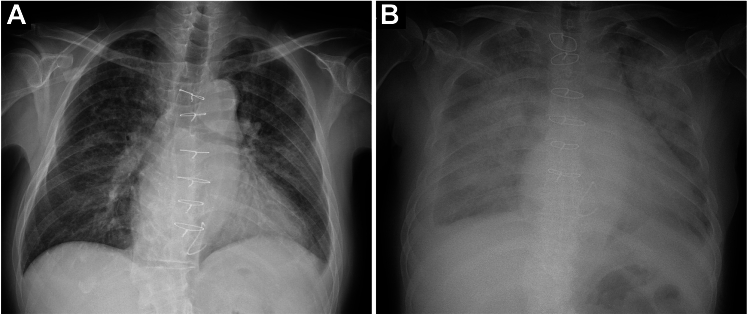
Chest Radiographs (A) At the initial visit, marked pulmonary congestion is visible. (B) After rapid decline, flash pulmonary edema is noted.
